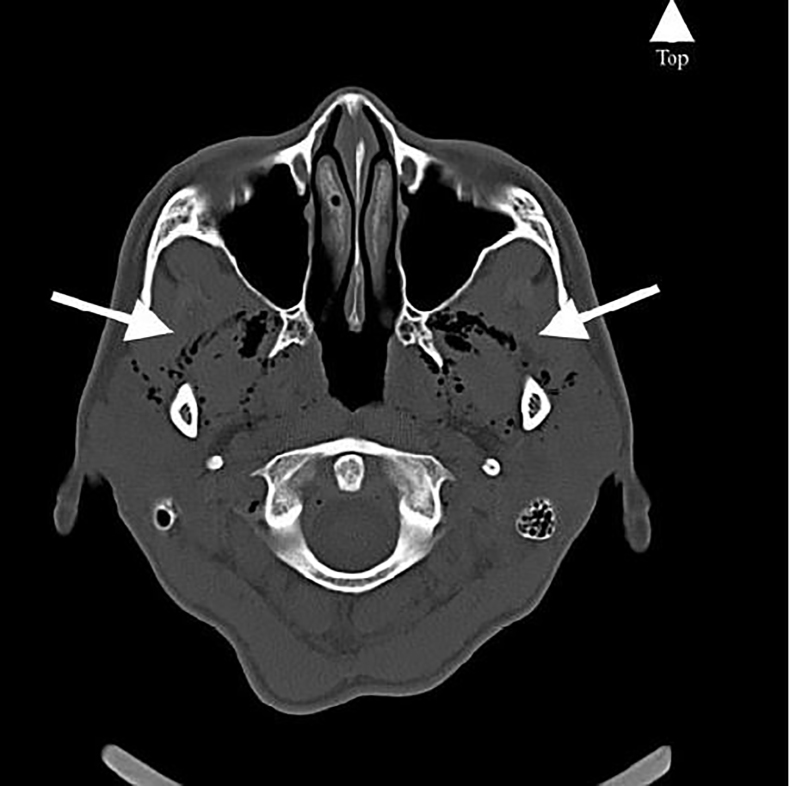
Axial computed tomography of the brain without contrast demonstrating bilateral air tracking into the pterygoid muscles (arrows).
